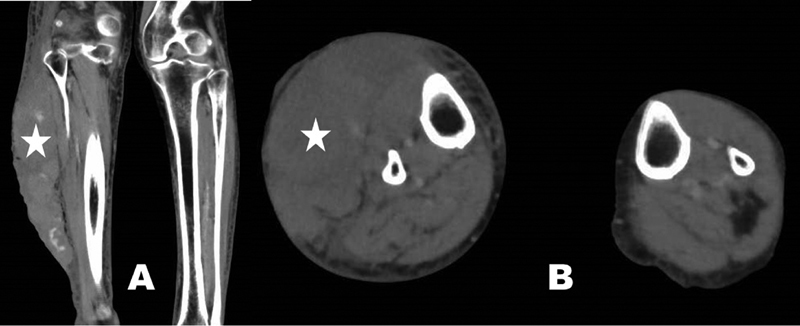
Contrast-enhanced computed tomography shows a large hematoma (indicated by the star) in the right calf 6 hours after trauma; (
